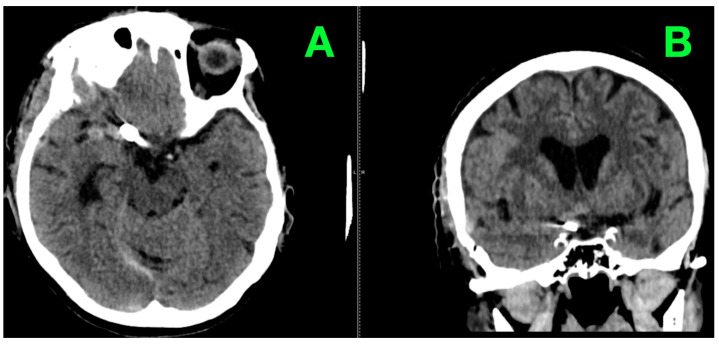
Postoperative non-contrast CT. (A): presents an axial view where the metallic artifact of the clip is clearly visible, verifying its proper positioning relative to the internal carotid artery. Surrounding structures demonstrated no evidence of residual or new hemorrhage, and there were visible signs of subarachnoid blood resorption within the basal cisterns. (B): a coronal reconstruction provides additional confirmation of the clip’s alignment at the hypophyseal segment of the internal carotid artery. The cerebroventricular system remained normal, with no evidence of midline shift, hydrocephalus, or other abnormalities. The basal cisterns appeared clearer, reflecting ongoing resolution of the subarachnoid hemorrhage.
